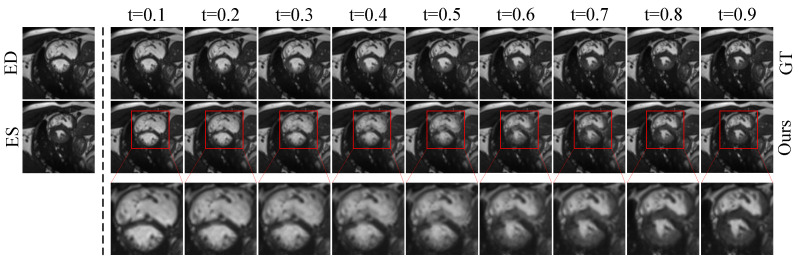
Visualization of interpolated cardiac MRI frames at arbitrary time points.
